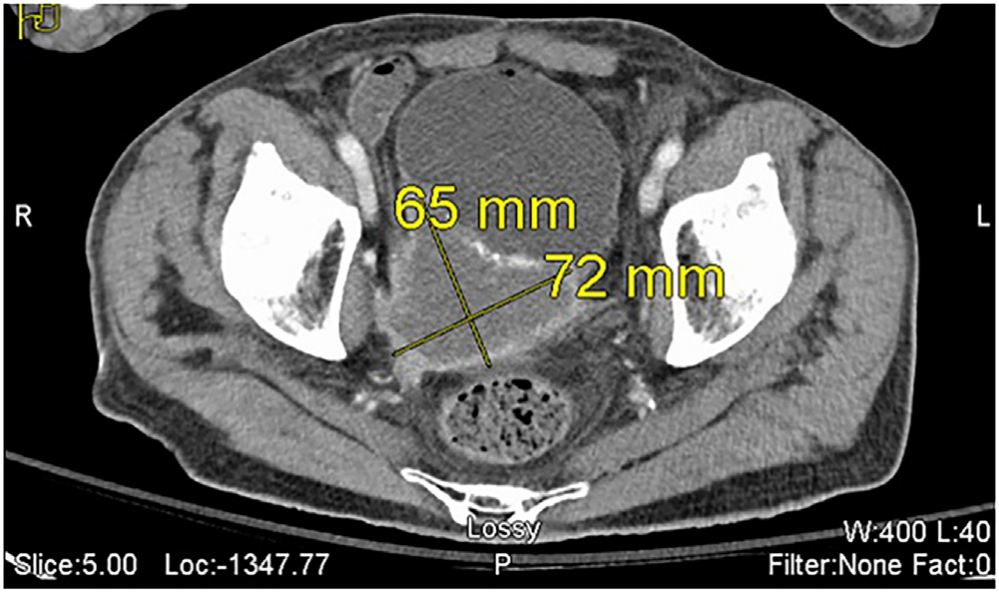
Axial CT views of the urothelial carcinoma 4 months after transurethral resection. The irregular mass has more than doubled in size to 65 × 72 mm and is in the same region as the previously resected urothelial cancer, suggesting likely recurrence.
